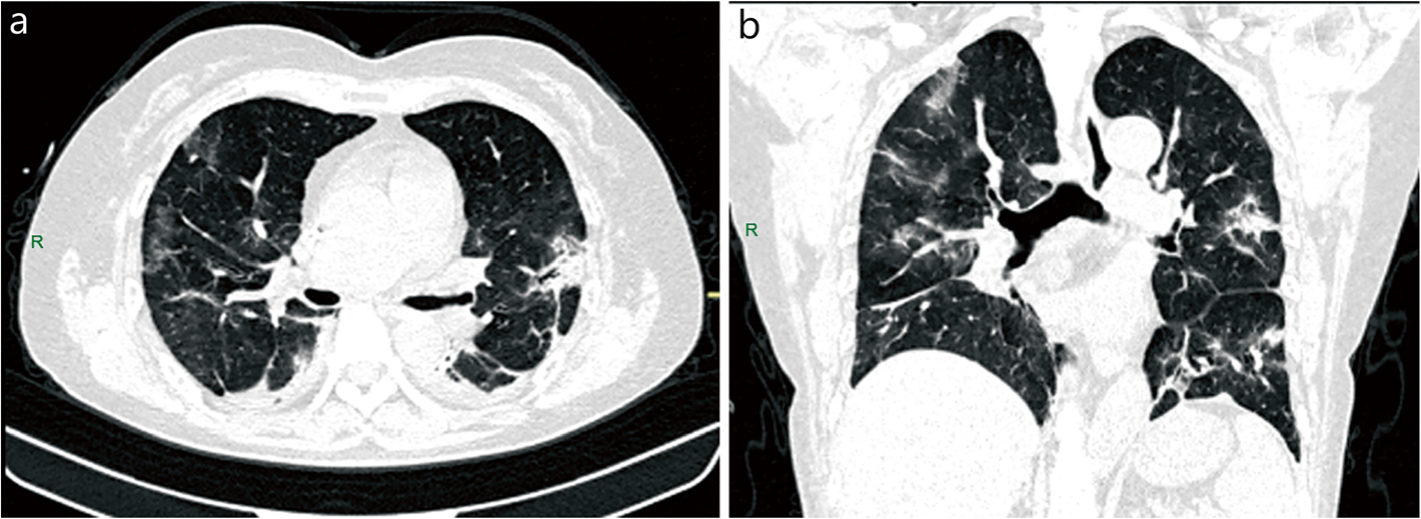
Chest CT of critical patient. A 58-year-old female patient with intermittent fever, cough and sputum for more than 1 week. Multiple patchy ground-glass opacities were seen in both lungs, and air bronchogram in the left upper lobe. Axial (a) and coronal (b) sections in lung windows. R: right
